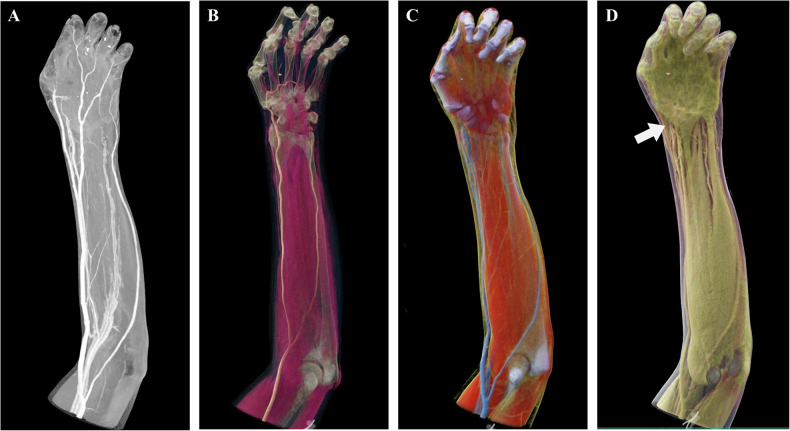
Palmar scar contracture with motion limitation in a 44-year-old male. A The CTA-based MIP image reveals occlusion of the distal right ulnar artery, its branches, and the distal branches of the deep palmar arch. B–D CR images, through adjustments in opacity and lighting, not only depict vascular pathologies but also realistically reconstruct the muscular anatomy. These images clearly demonstrate atrophy and collapse of the thenar muscles corresponding to the area of vascular occlusion, visually elucidating the causal relationship between vascular pathology and muscle atrophy; the arrow in D specifically indicates the significant atrophy and collapse of the thenar muscles. This approach achieves an integrated “vessel-end organ” comprehensive assessment
